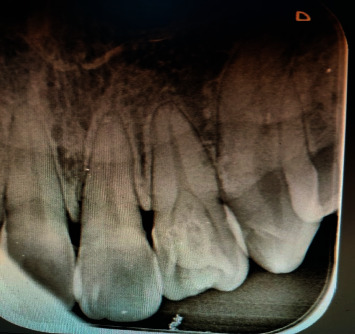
Intraoral periapical radiograph showing talon's cusp with dens invaginatus in maxillary left lateral incisor.
